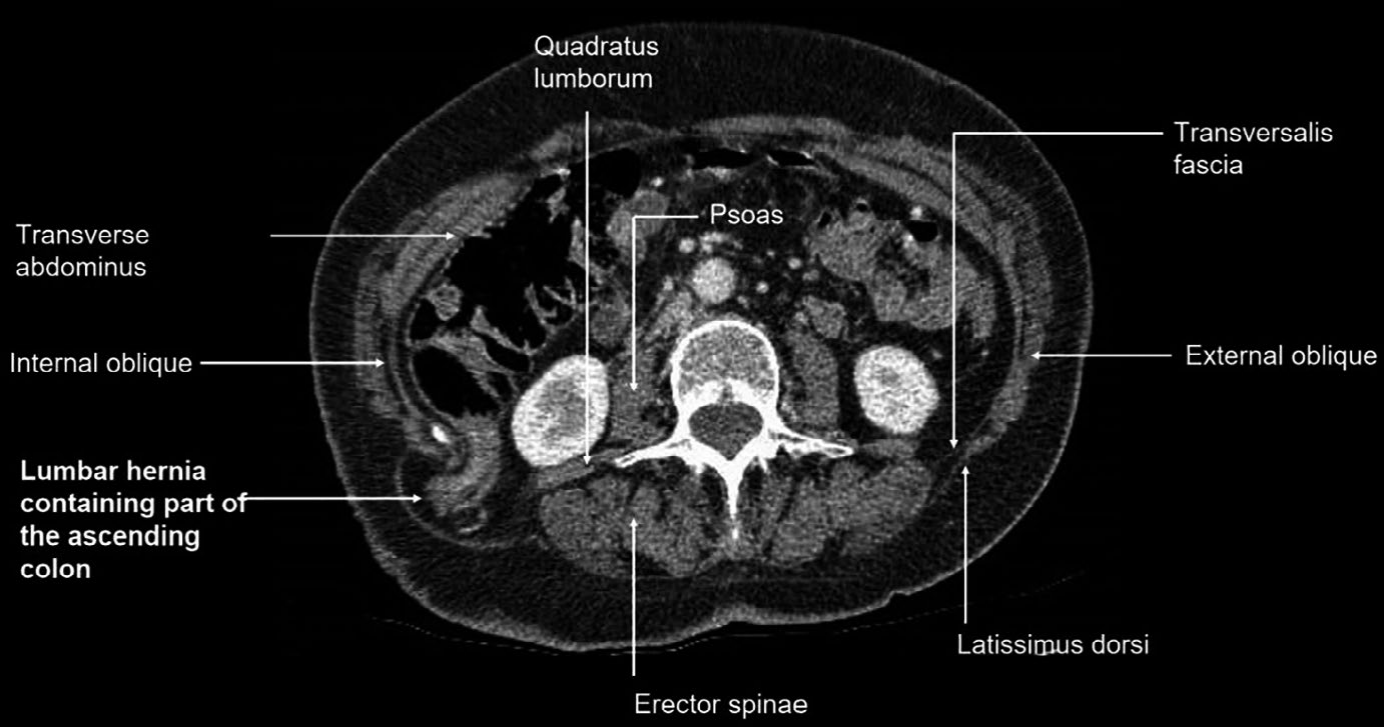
Transverse slice of CT abdomen showing ascending colon in hernial sac
